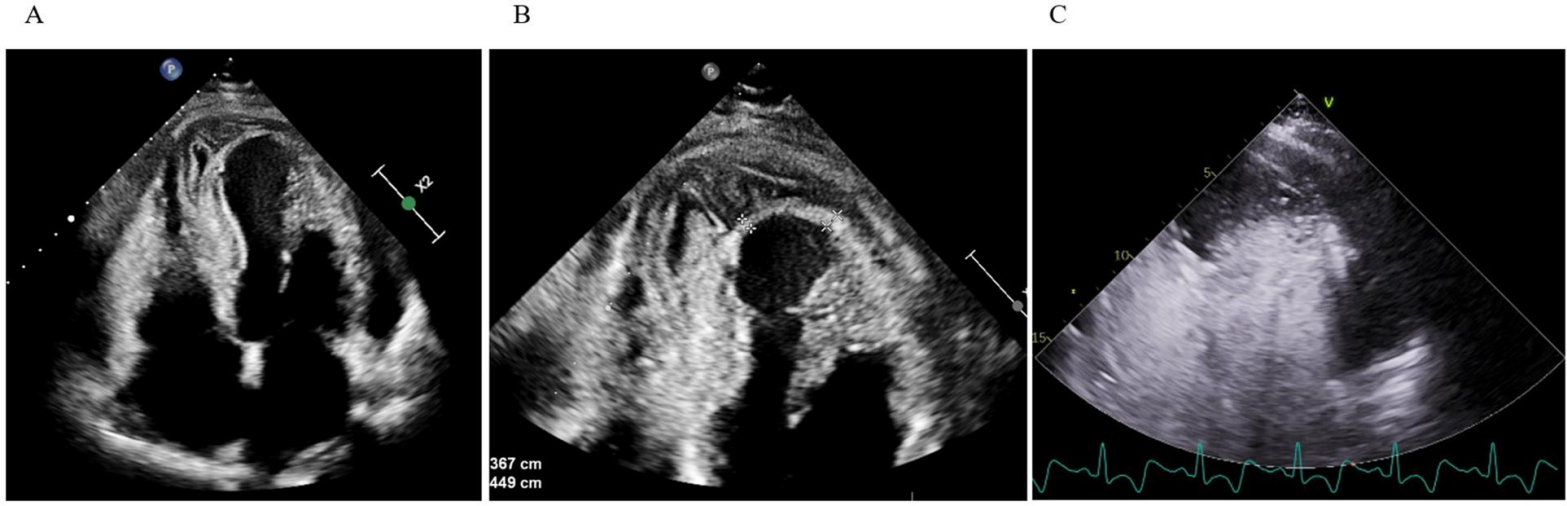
Transthoracic echocardiography. (A,B) Apical four-chamber view shows apical and inferoseptal myocardial thickening with a large, echolucent, spindle-shaped intramyocardial cavity, without evident communication to cardiac chambers or pericardial space. (C) Contrast echocardiography demonstrates no flow from the ventricular cavity into the lesion.
